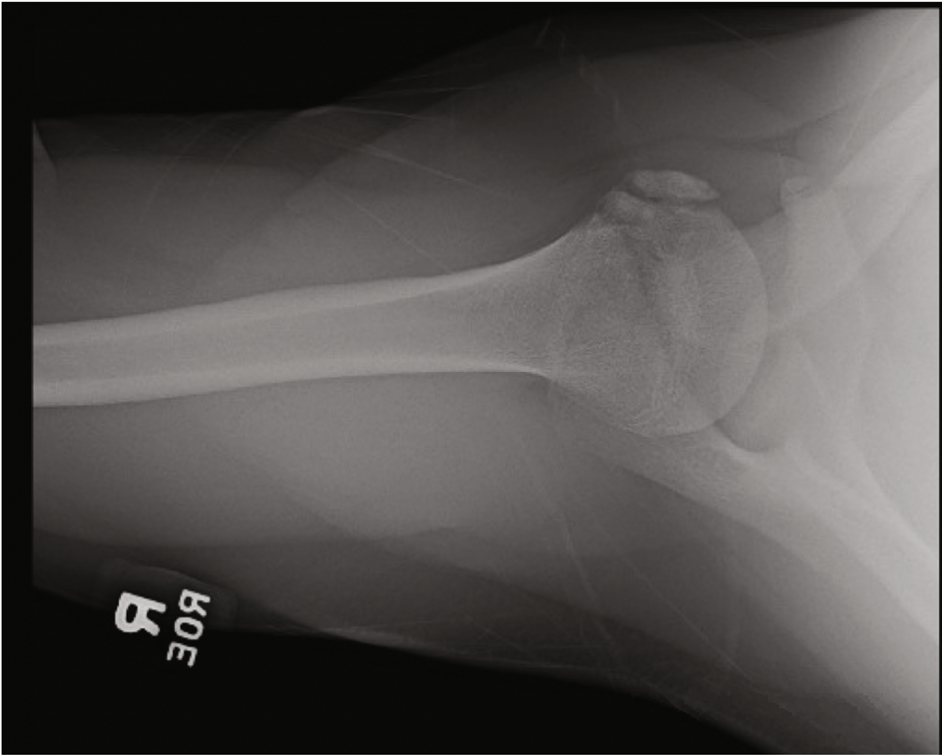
Axillary X-ray reveals age indeterminate fracture from the humeral head at the time of outpatient follow-up one year after injury.
